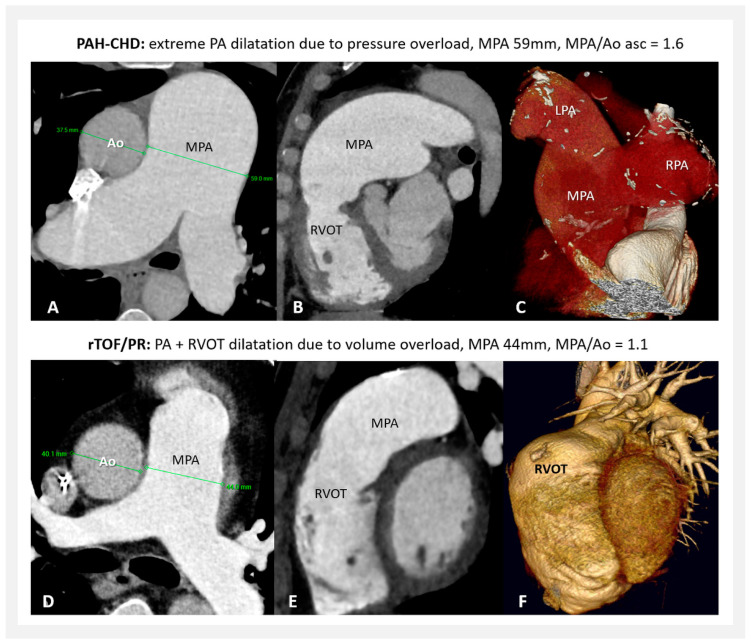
(A–C) MPA dilatation in PAH-CHD (CT); (D–F) MPA plus RVOT dilatation in rTOF/PR (CT). PAH-CHD—pulmonary arterial hypertension associated with congenital heart defects; rTOF/PR—repaired Tetralogy of Fallot with isolated severe pulmonary regurgitation; PA—pulmonary artery; MPA—main pulmonary artery; RVOT—right ventricular outflow tract; LPA—left pulmonary artery; RPA—right pulmonary artery; Ao—aorta; and CT—computer tomography.
